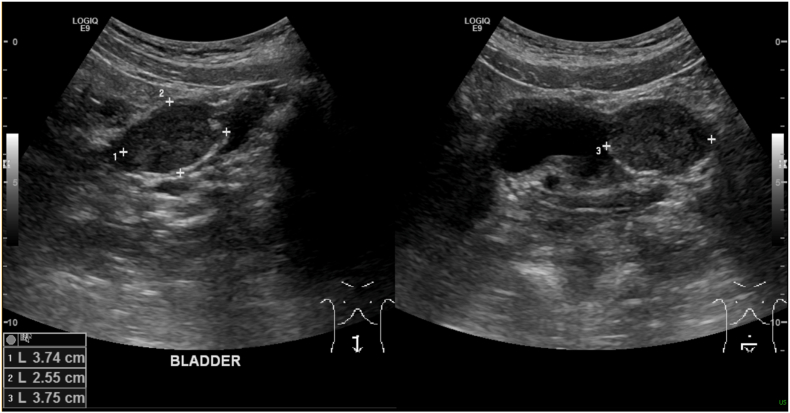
Legend: Transabdominal bladder ultrasonography demonstrating a well-defined, heterogeneous mass measuring 3.8 x 3.7 × 2.6 cm is noted at the left ureterovesical junction. The lesion was initially suspicious for an intravesical thrombus. Associated left-sided hydroureter is visualized superior to the mass.
